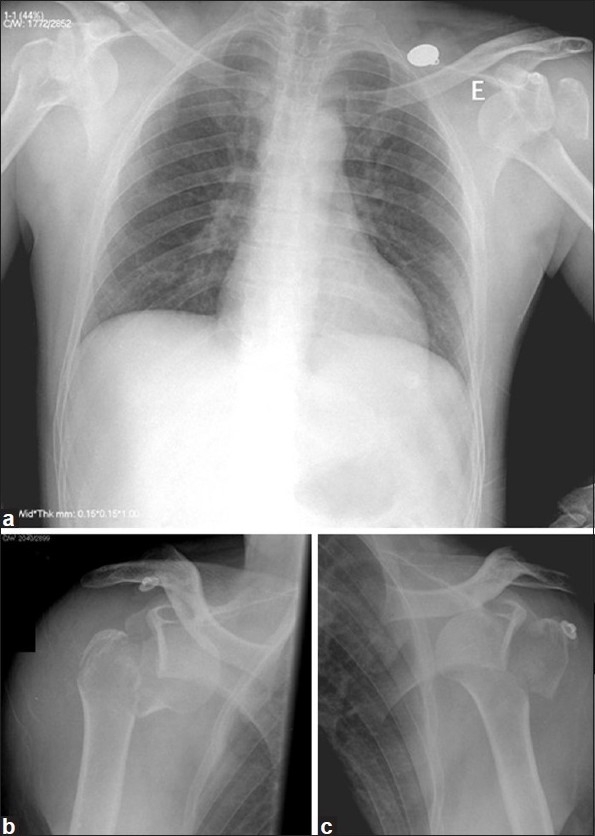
Radiographs of both shoulders (a) showing a posterior four-part fracture-dislocation of the right shoulder (b) and a posterior two-part fracture-dislocation of the left shoulder (c)
